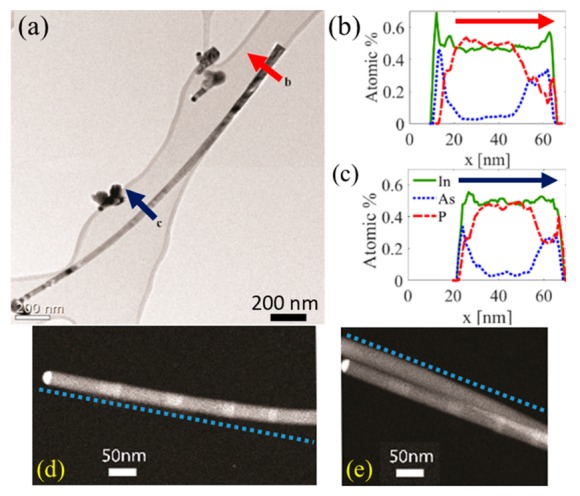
(a) TEM image of a bent InP–InAs heterostructure NW. (b,c) Energy-dispersive X-ray (EDX) analysis of the wire cross section at (b) wire base and (c) wire middle. Arrows show scan direction. (d,e) High-resolution TEM (HRTEM) scans of NW heterostructures showing further bending in addition to the creation of axial InAs segments. Dashed lines are a guide to the eye.
